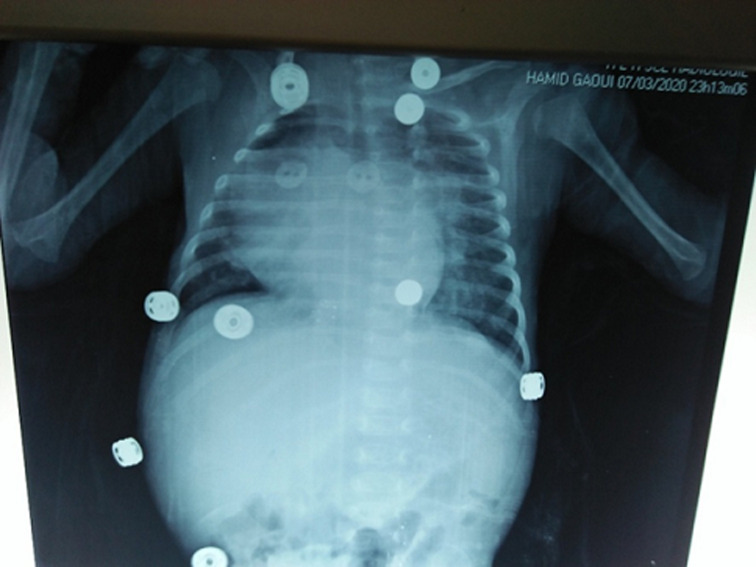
frontal thoracoabdominal radiograph: cardiomegaly
